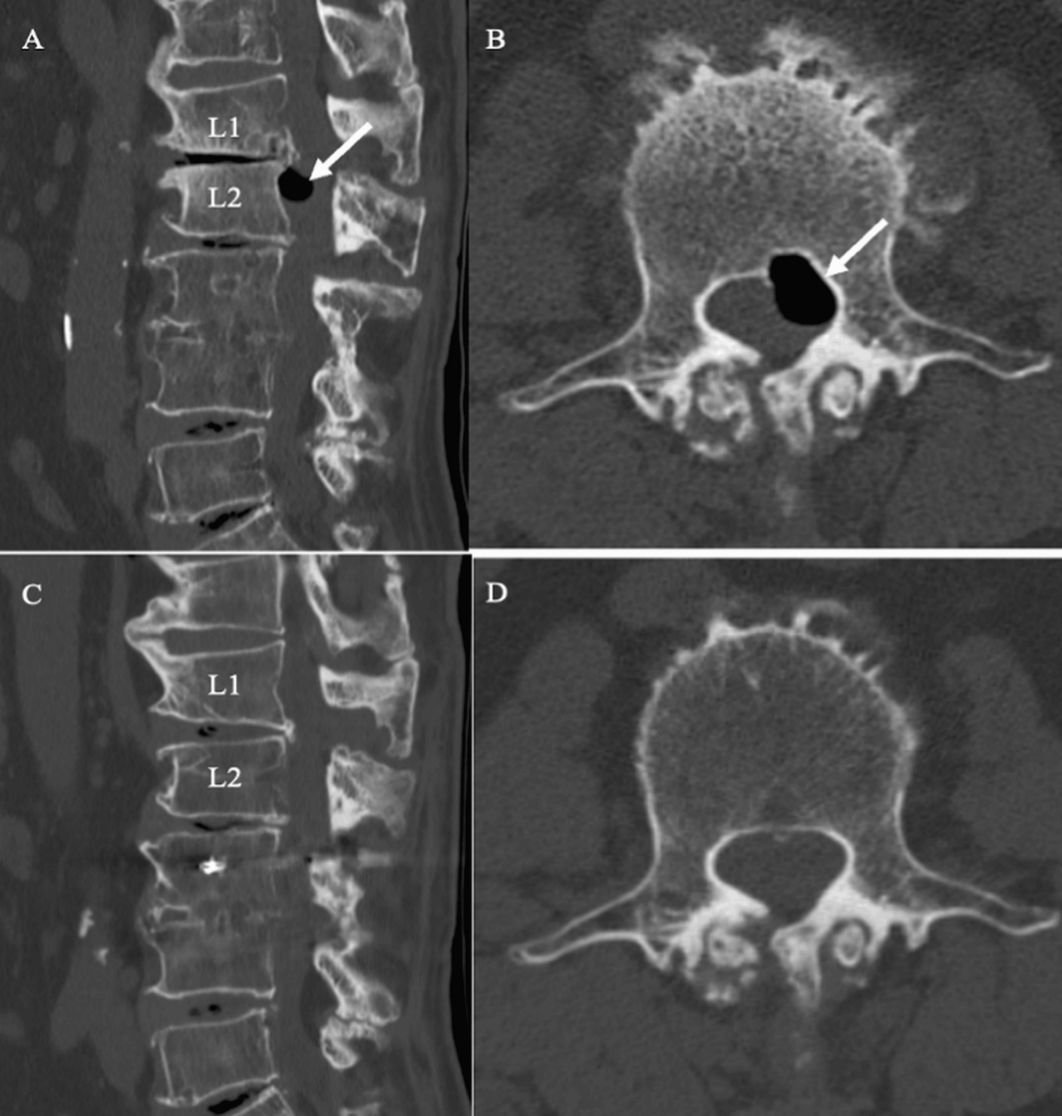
Preoperative CT Demonstrating Vertebral Scalloping and Epidural Gas Accumulation Sagittal (A) and axial (B) CT images obtained 18 days before surgery show an epidural gas-containing cyst causing posterior scalloping of the L2 vertebral body *(white arrow)*. For comparison, sagittal (C) and axial (D) CT images obtained 18 months earlier show no significant scalloping at that time.
